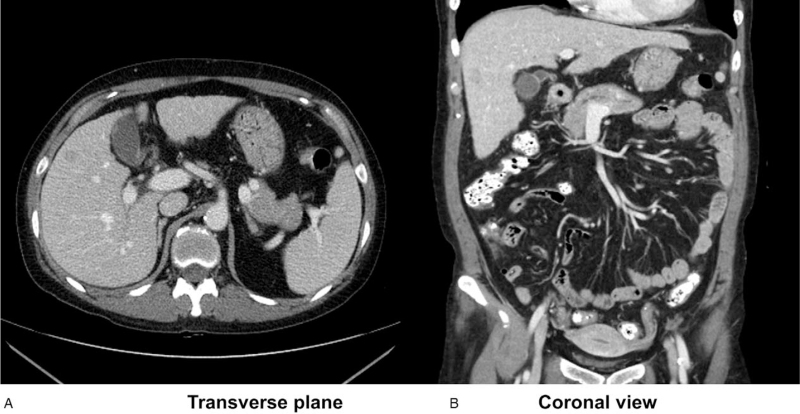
Computed tomography (CT) of the patient. (A) Transverse plane, (B) coronal view. Contrast-enhanced abdominal CT revealed liver cirrhosis and there was no significant change of about 1.3 cm sized enhancing hepatic nodule on right hepatic lobe which is pathologically proven metastatic renal cell carcinoma. There was no obstructive lesion on biliary tract.
